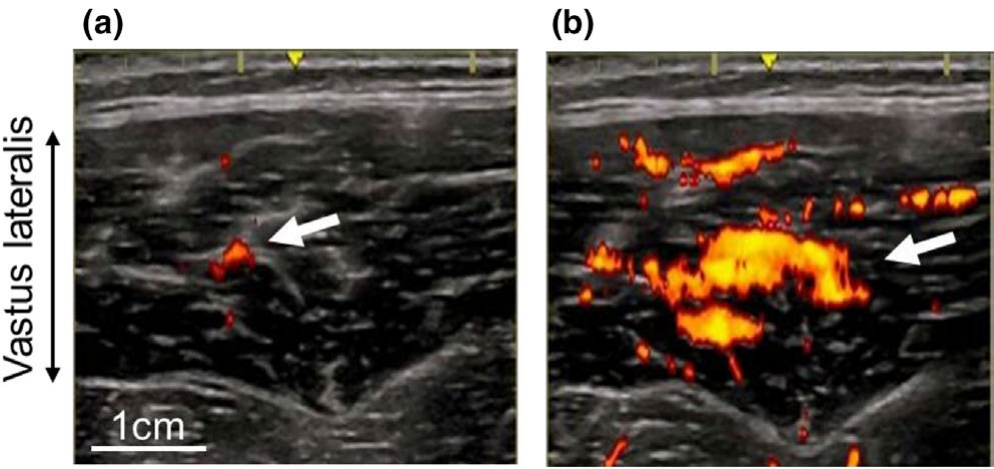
Representative power Doppler images within the middle portion of the vastus lateralis at baseline (a) and immediately after exercise (b). The arrows show power Doppler signals which indicate blood flow distribution.
